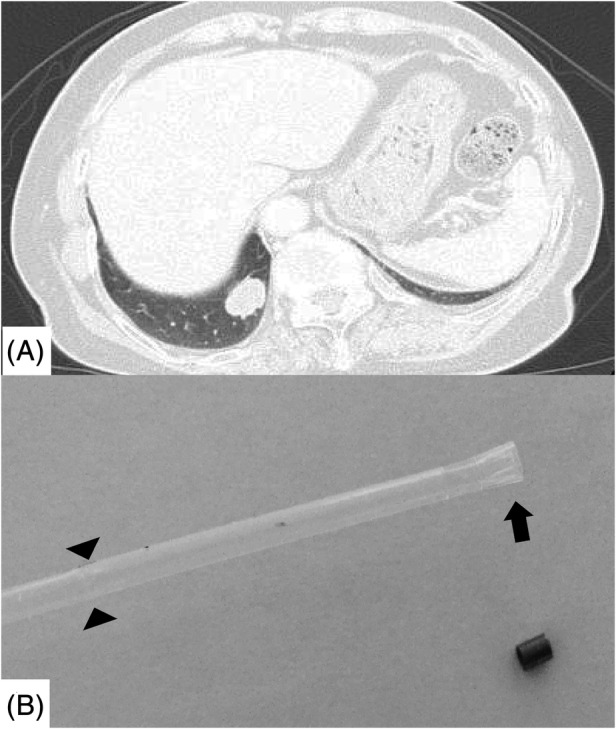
(A) Computed tomography revealing a solid mass of 39 mm in diameter in the right S10 region. (B) The head of the broken guide sheath was expanded (arrow). Traces of buckling were found near the head of the guide sheath (arrow heads).
